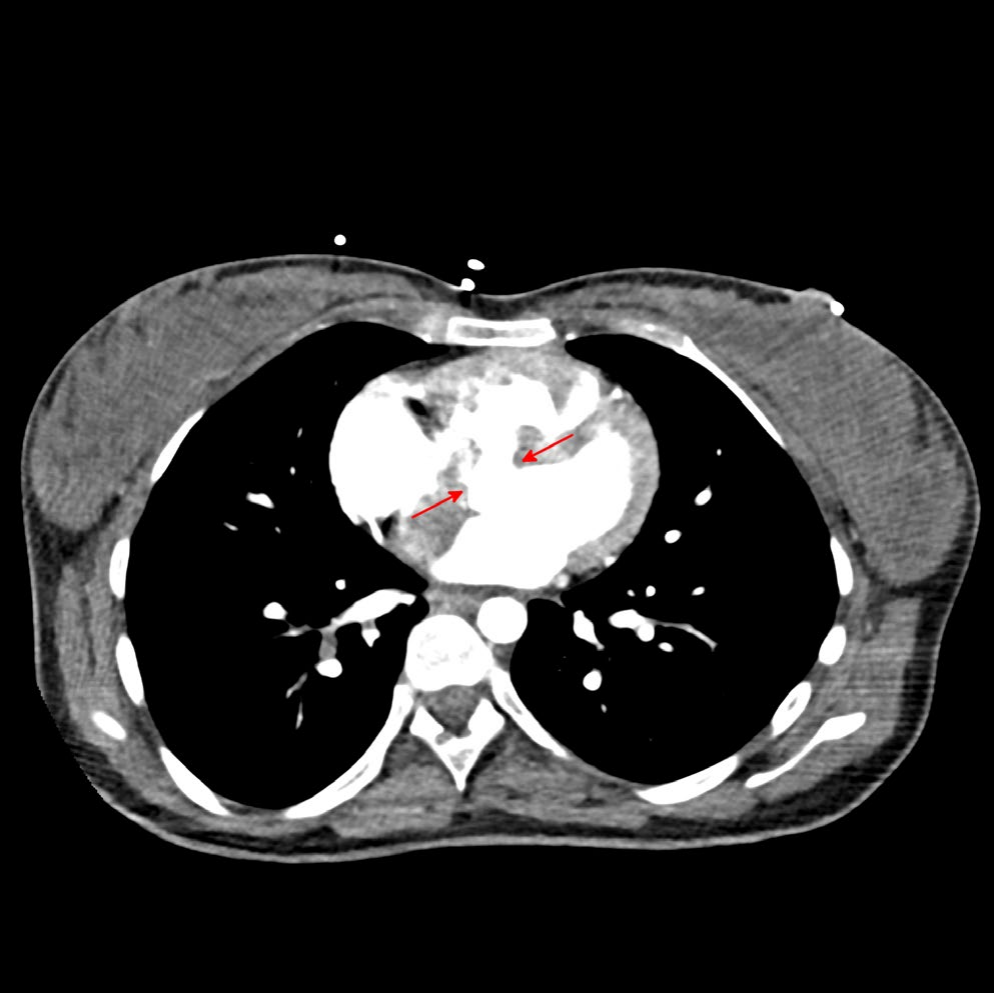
Exemplary transversal image from contrast medium enhanced thoracic computed tomography; large ventricular septal defect with shunt between the left and right ventricular outflow tract indicated by red arrows
